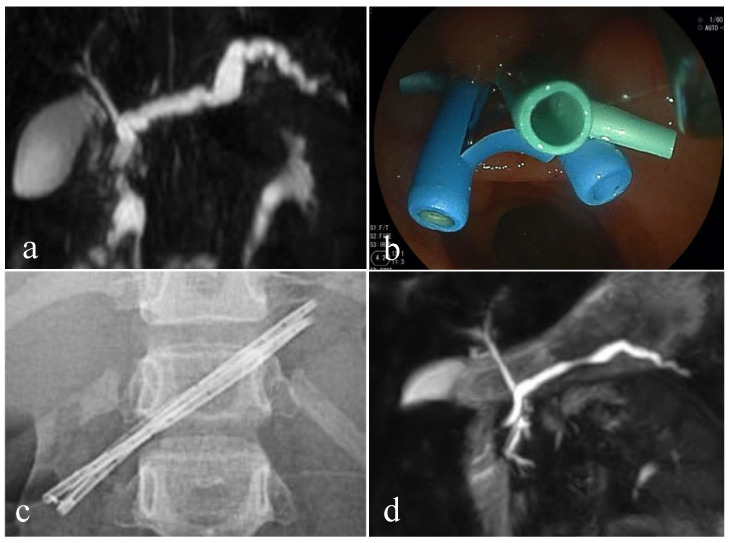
Multiple plastic stent placement therapy for children with hereditary pancreatitis. Magnetic resonance cholangiopancreatography (MRCP) before endoscopic treatment. The main pancreatic duct is meandering and markedly dilated (a). Endoscopic view of placement of 3 plastic stents from the minor papilla (b). Abdominal X-ray shows 3 plastic stents placed in the main pancreatic duct (c). MRCP 16 months after plastic stent removal. Dilation of the main pancreatic duct is improved (d).
